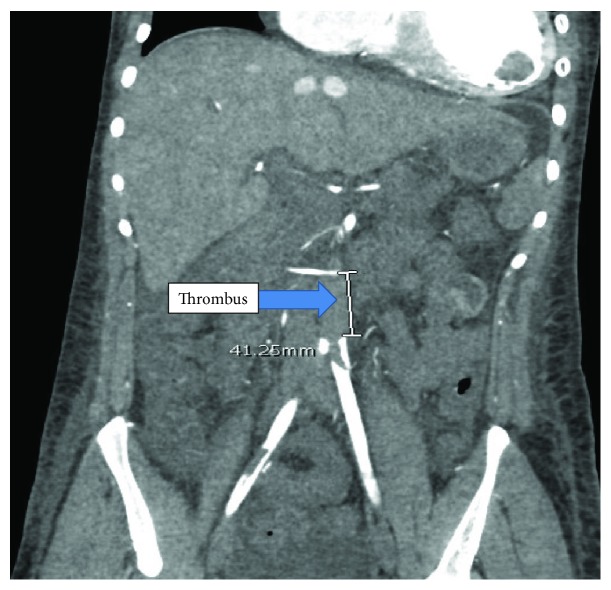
CT angiogram showing a massive AAA thrombus beyond the renal arteries.
